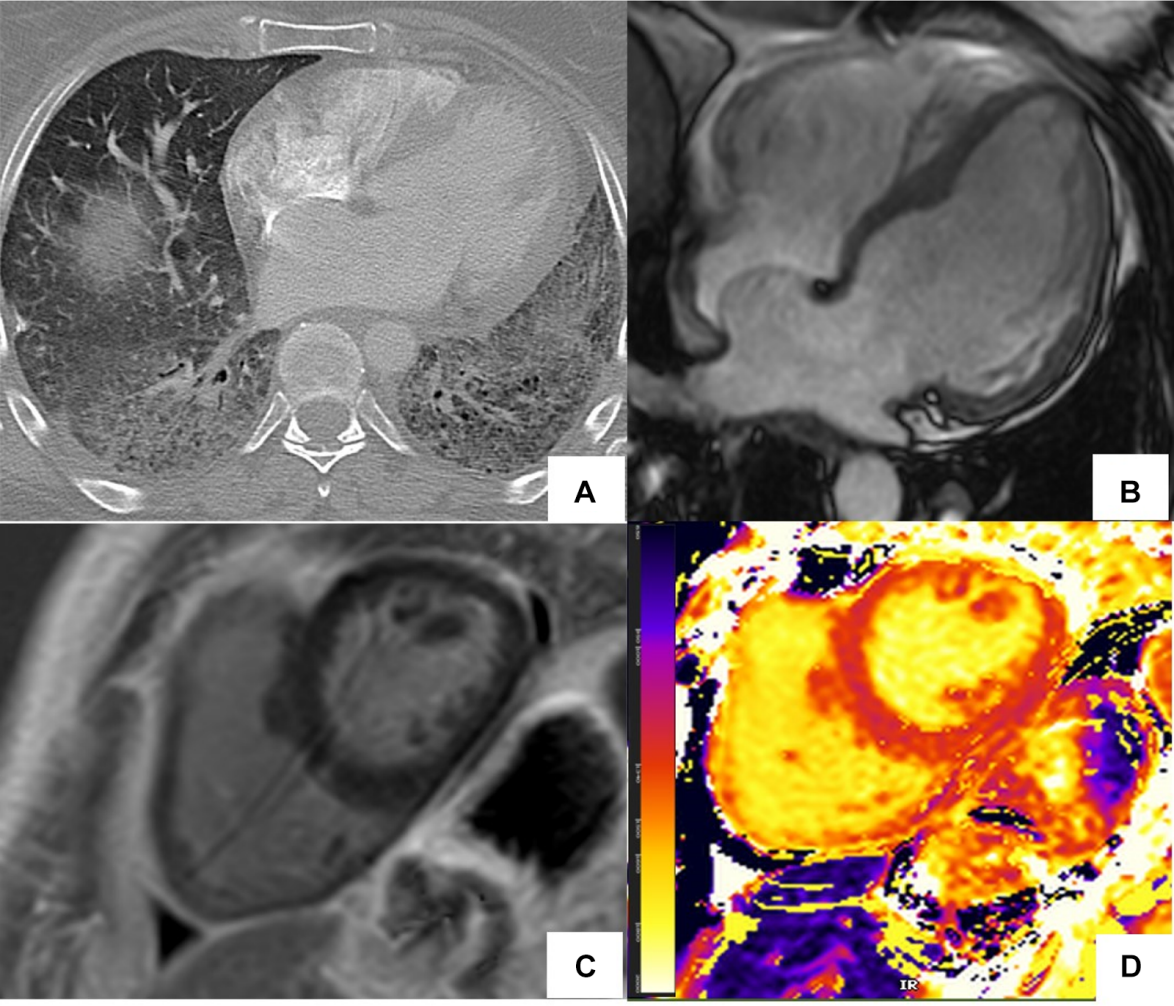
Abnormal cardiac MR (CMR) T1 mapping in subclinical myocardial inflammation in a patient with scleroderma. 37-year-old female with scleroderma-related interstitial lung disease (A Chest CT) and increasing dyspnea on exertion. 1.5 T cine CMR had a normal LV ejection fraction of 59% and no regional wall motion abnormality. 4ch cine showed flattening of the inter-ventricular septum consistent with known pulmonary hypertension (B) On short-axis late gadolinium enhancement phase-sensitive inversion recovery (LGE-PSIR) there was no myocardial late gadolinium enhancement, only trace pericardial effusion (C) Native T1 values were elevated to 1,250 ms on the native T1 color map (D) suggesting active myocardial inflammation.
